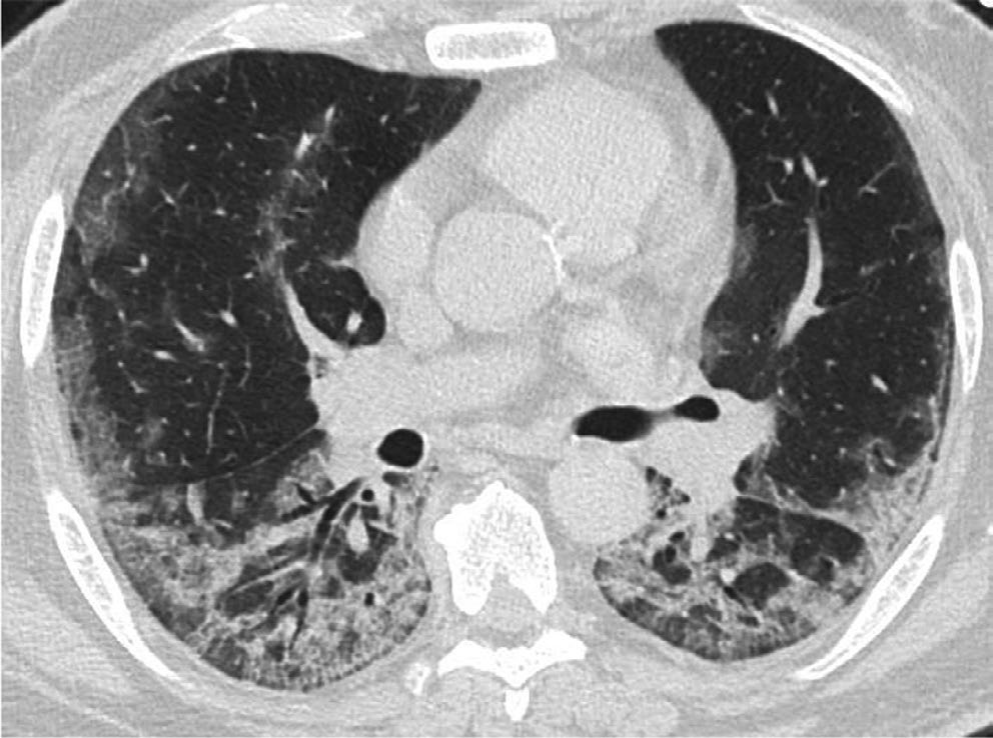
Chest computed tomography on admission to the referring hospital. Ground‐glass opacities and infiltrative shadows were observed in the lower lobes of the bilateral lungs with a subpleural predominance
